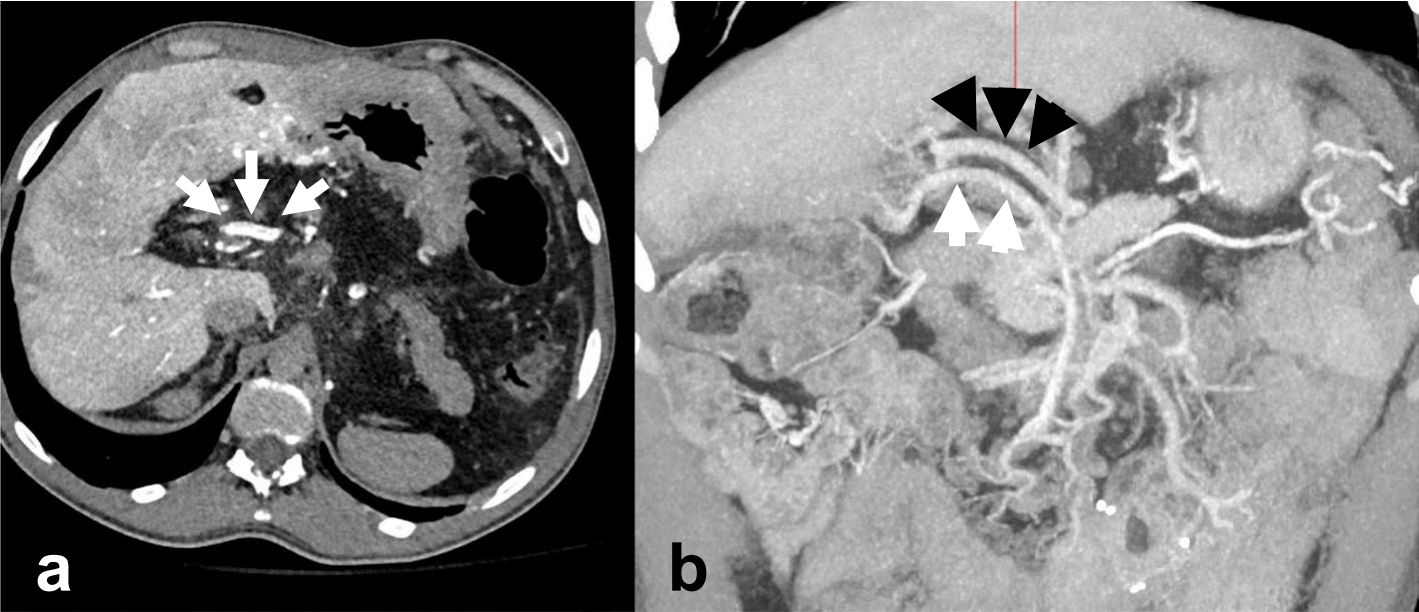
A CT arterioportography axial image with contrast injected via an SMA catheter showed (a) known chronic portal vein thrombus and prominent SMV collateral in the porta hepatis (white arrows). A coronal image from multiplanar reformatted maximum intensity projections shows the relationship of the HA (black arrows) relative to the dominant collateral (white arrows)
